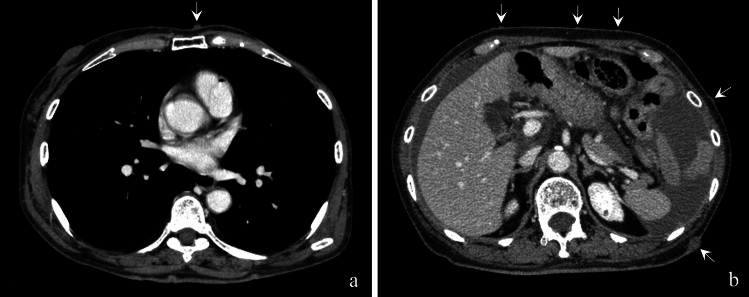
(a) Contrast chest CT scan (b) Contrast abdominal CT scan
